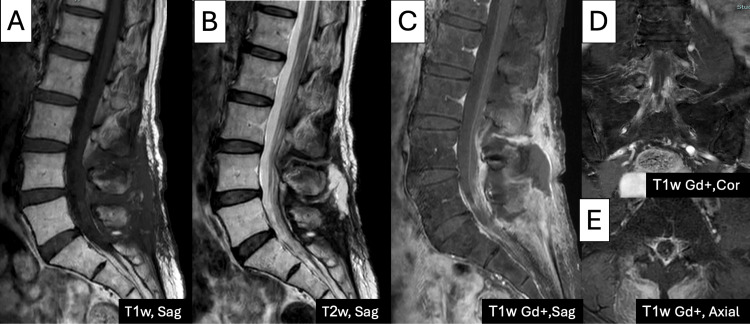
An MRI obtained nine months after surgery showed that the extradural intramedullary tumor was completely removed on the sagittal plane on T1-weighted (T1W) image (A), on T2-weighted (T2W) image (B), and after contrast injection with the sagittal plane (C), coronal plane (D), and axial plane (E).
